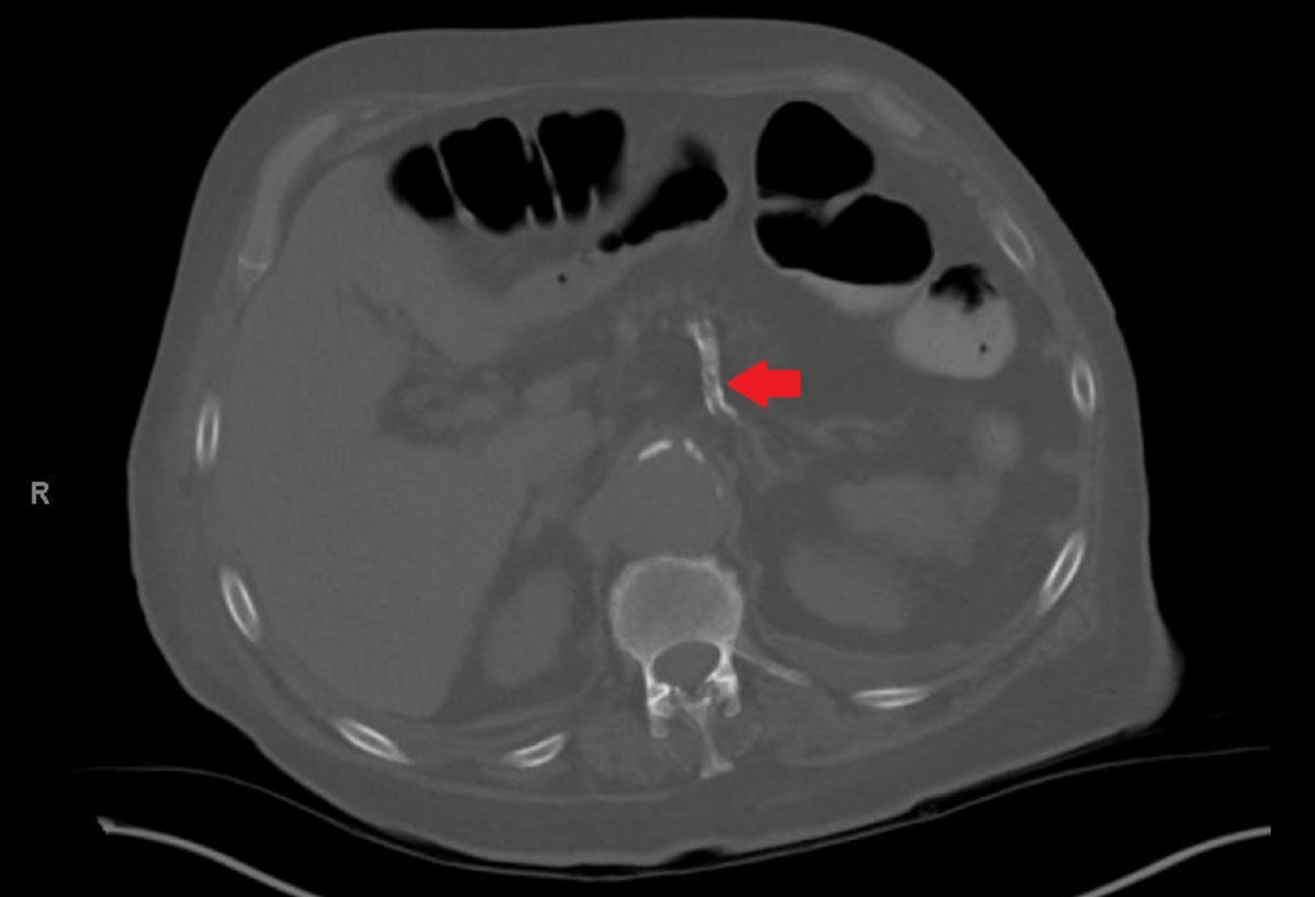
CT abdomen and pelvis with oral and rectal contrast showing fistulation between the sigmoid colon and the bladder (red arrow). CT: computed tomography
